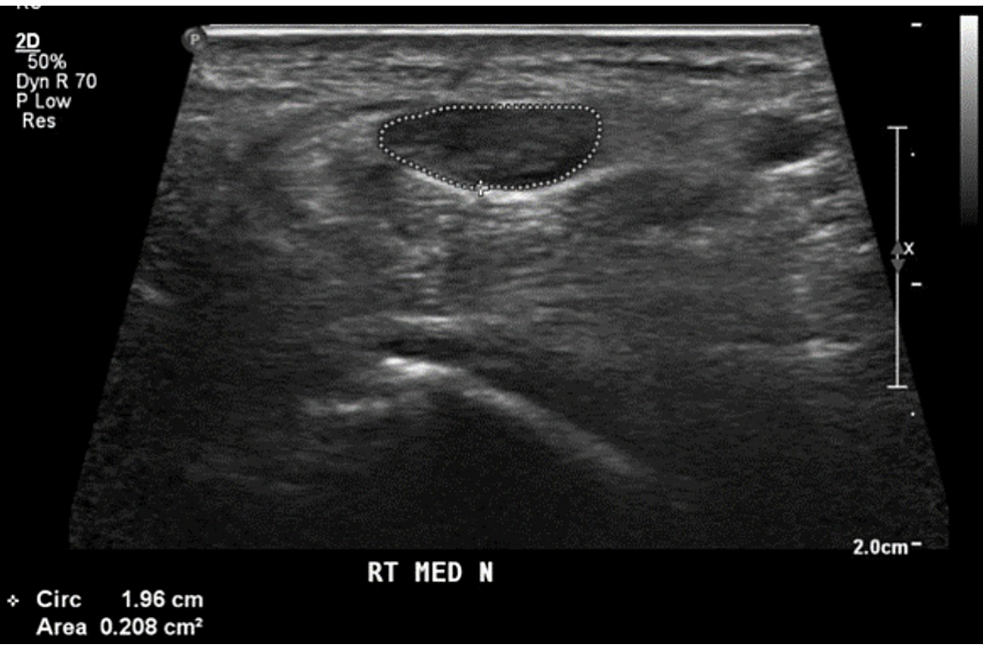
A transverse ultrasound view of the right wrist demonstrates the encircled surface area of the entrapped right median nerve with a cross-sectional area measuring 21 mm (2) representing severe carpal tunnel syndrome.
